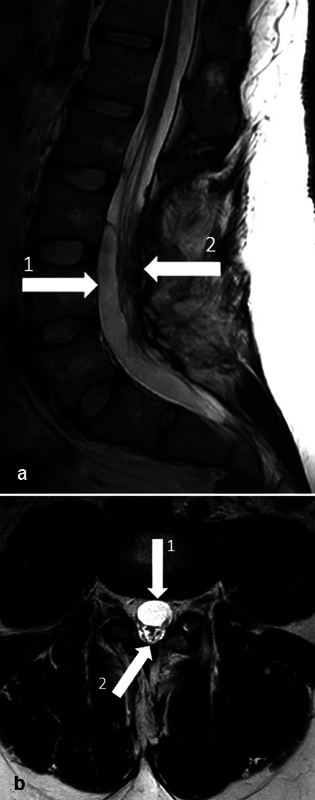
Preoperative magnetic resonance imaging (MRI) of lumbosacral spine. (
